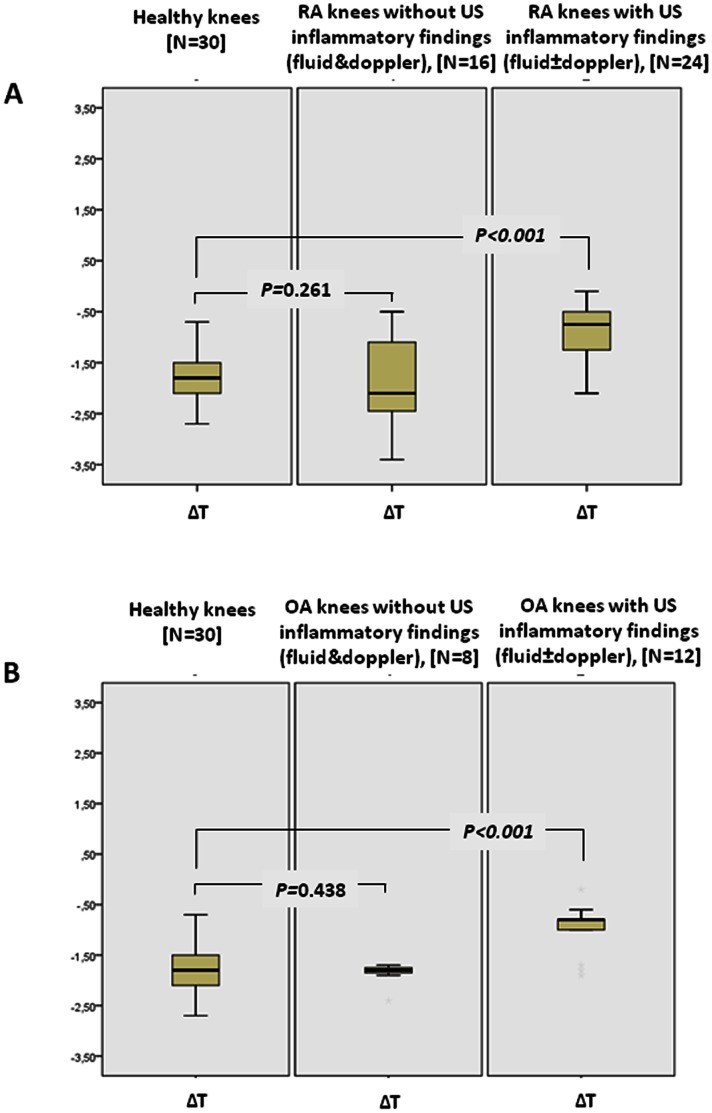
Temperature differences (ΔT) recorded by microwave radiometry device. Temperature differences (ΔT) between knee joint and thigh (control point) of healthy subjects and (A) patients with rheumatoid arthritis (RA) or (B) patients with osteoarthritis (OA) stratified by the presence of excess fluid and/or Doppler signal evidenced by knee joint ultrasound (US). Patients’ knee joints with inflammatory US findings had smaller ΔT compared to healthy knees and patients’ knees with normal US findings.
